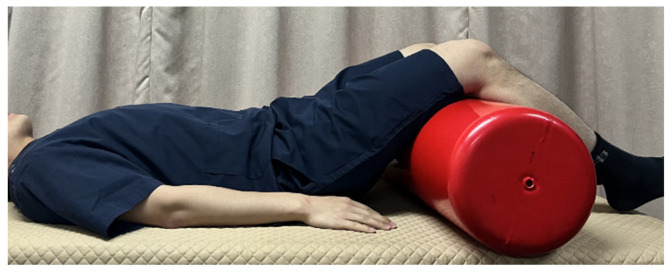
Crook-lying position for ultrasound imaging of the abdominal muscles.
